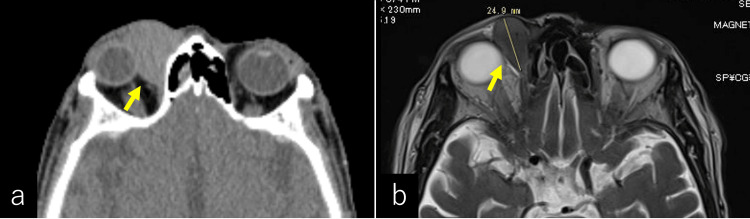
Imaging findings of the right upper eyelid mass at initial diagnosis Computed tomography imaging (a) revealed a well-defined mass, displacing the right eyeball laterally, with bilateral cervical lymphadenopathy. No other definitive tumors or nodules were identified. T2-weighted imaging (T2WI) MRI (b) showed a smooth-edged nodule in the medial right orbit, measuring 24.9 × 21.5 × 30.5 mm in size, with mildly hyperintense signal characteristics.
